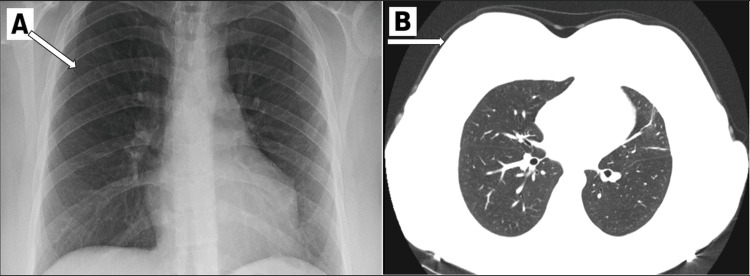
Chest radiography and computed tomography imaging. (A) Frontal chest radiograph showing no focal consolidation or acute cardiopulmonary abnormality. (B) Axial inspiratory lung-window computed tomography image showing clear lung parenchyma without emphysema, bronchiectasis, consolidation, focal nodularity, or interstitial abnormality. Dynamic airway assessment in the full study showed no evidence of tracheomalacia.
